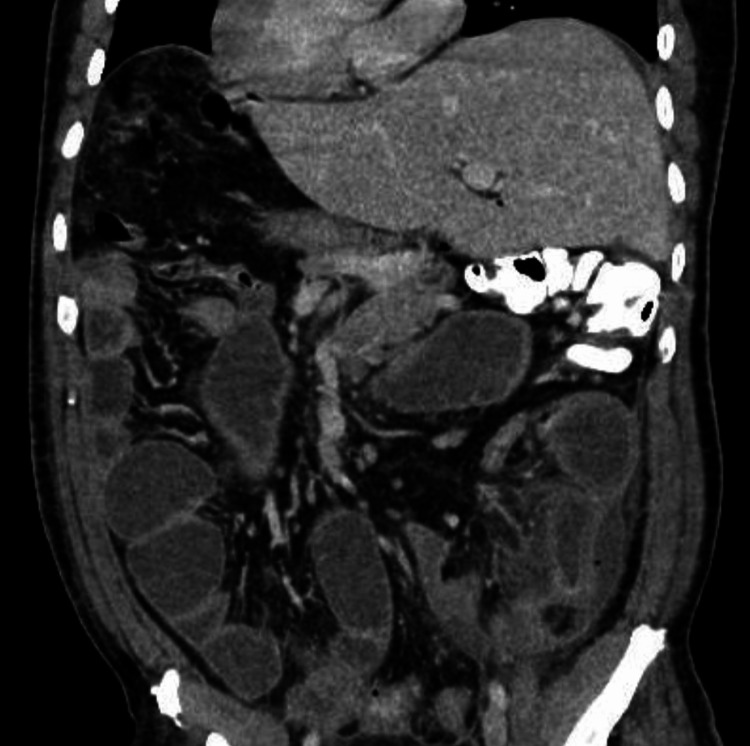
Coronal contrast-enhanced CT image demonstrating segmental small-bowel wall thickening in the left lower quadrant with marked adjacent mesenteric fat stranding, findings consistent with phlegmonous inflammatory change, suggestive of an underlying inflammatory etiology.
